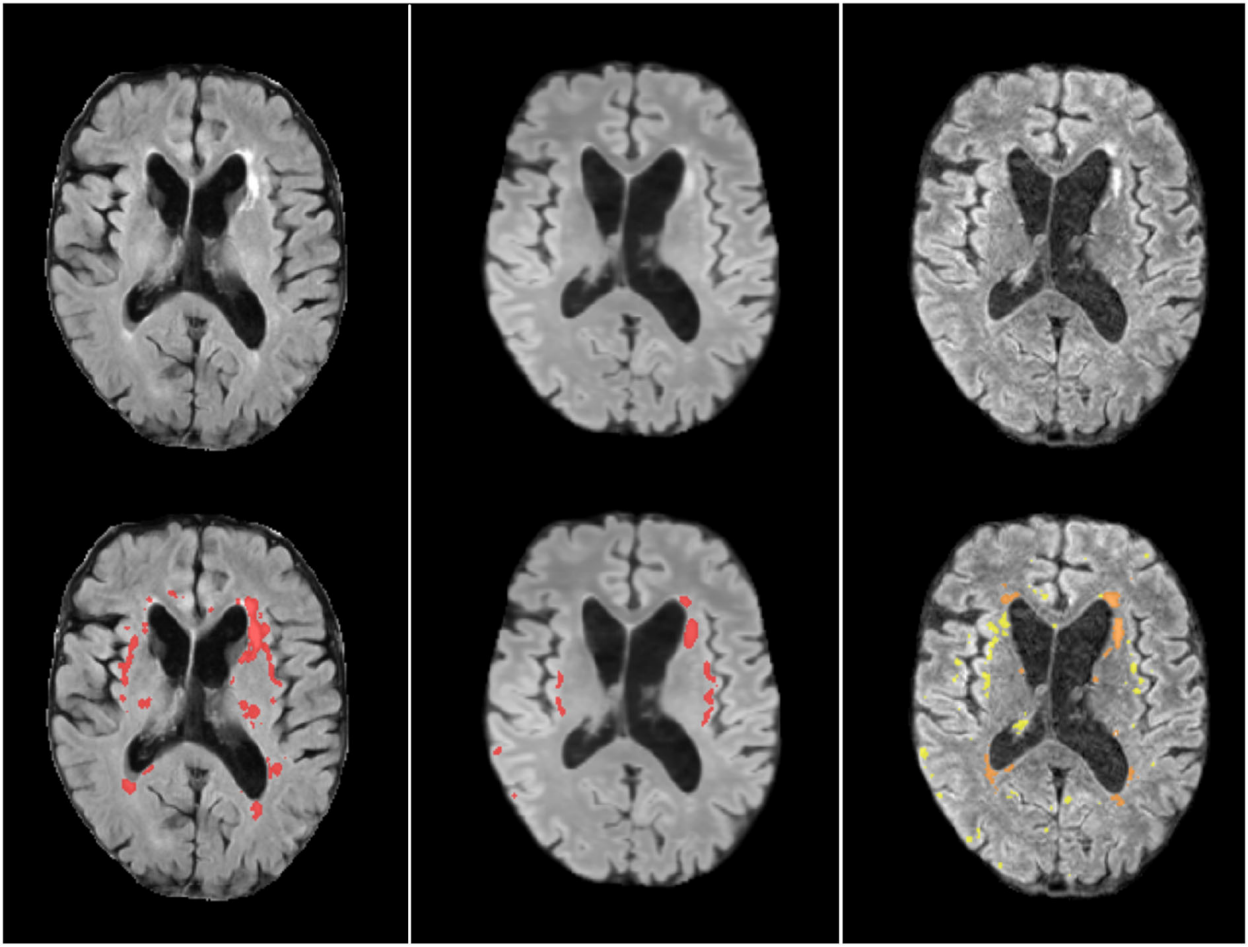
Axial FLAIR slice of low resolution (left column) resampled and aligned to the high resolution image (right column) using trilinear interpolation, and the correspondent SR image (center column). The bottom row shows the automatically generated binary masks of WMH superimposed in red for the low resolution and SR images, and in yellow for the high resolution image. The manually corrected WMH mask is also superimposed in the high resolution image and can be appreciated in an orange tone.
